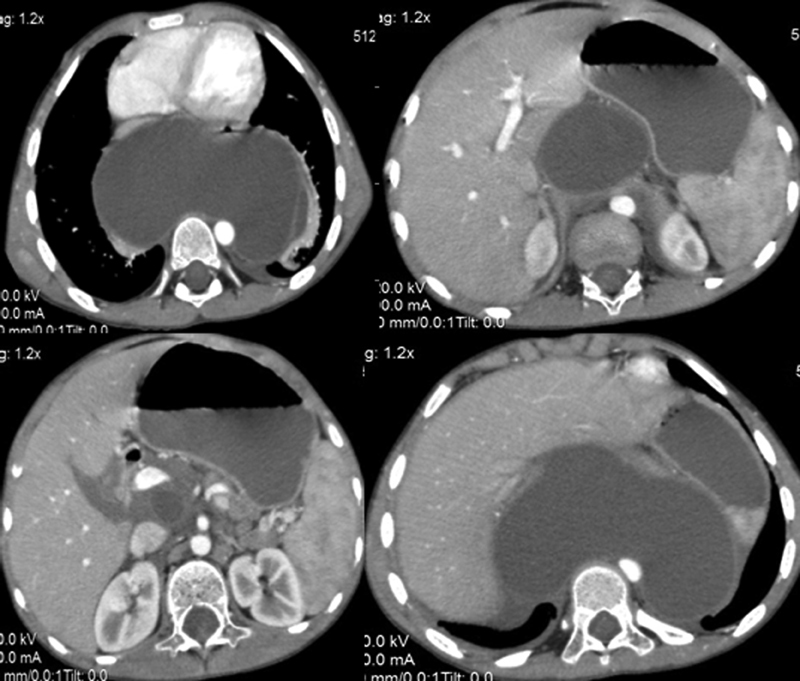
CT abdomen showing fluid containing cystic mass behind the stomach around the pancreas extending up to the chest. CT, computed tomography.
